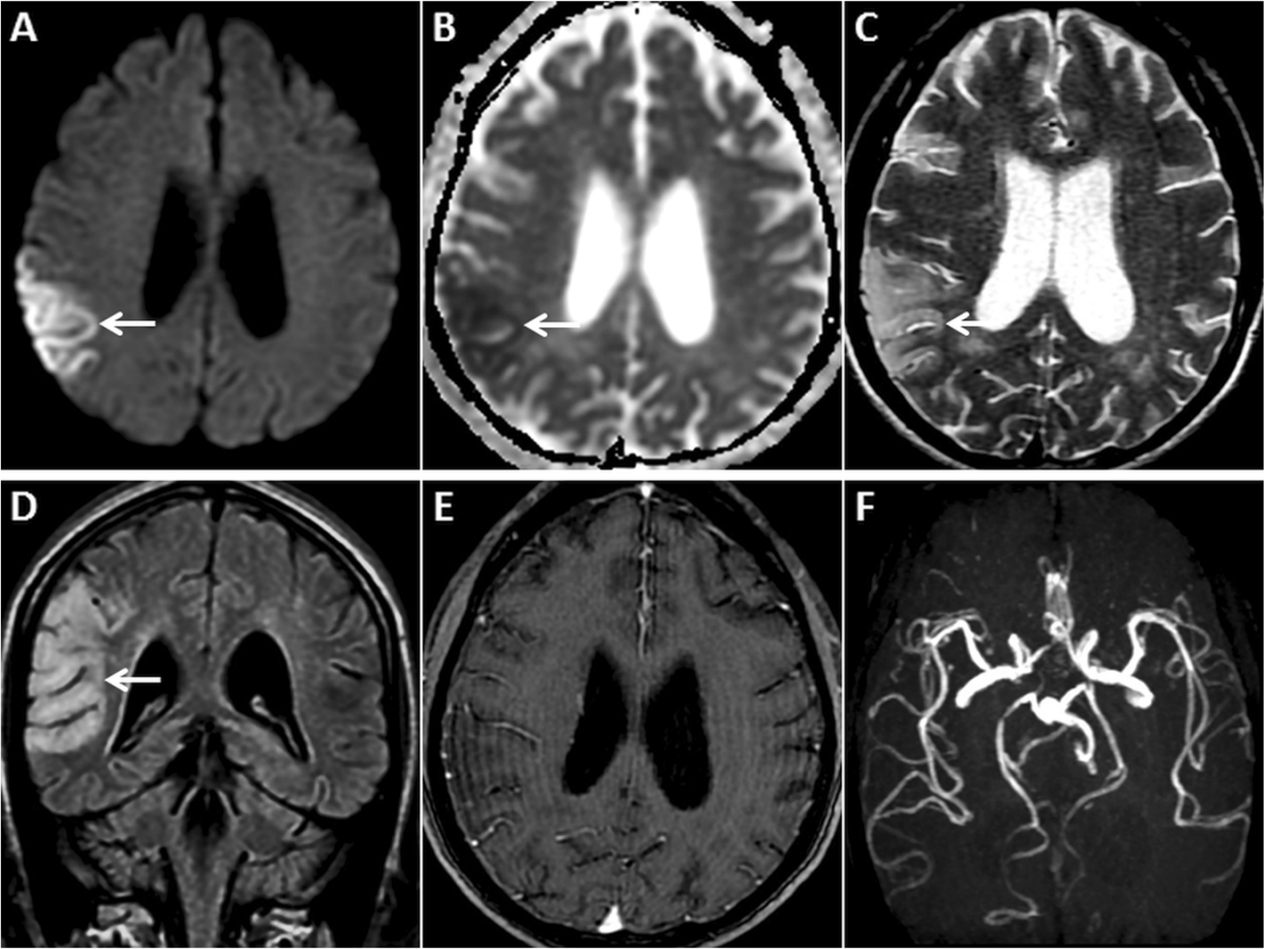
Pre- and post-contrast MRI of the brain in a 45-year-old male patient with proven MELAS on muscle biopsy. GRD is observed in the right parietal cortex (arrows) on axial DWI (a) and ADC image (b). Associated gyral swelling and hyperintense signal (arrows) is seen on axial T2W (c) and coronal FLAIR image (d). No enhancement is seen in this region on post-contrast axial T1W image (e). Reconstructed maximum intensity projection MRA image of Circle of Willis (f) confirms no large vessel occlusion
